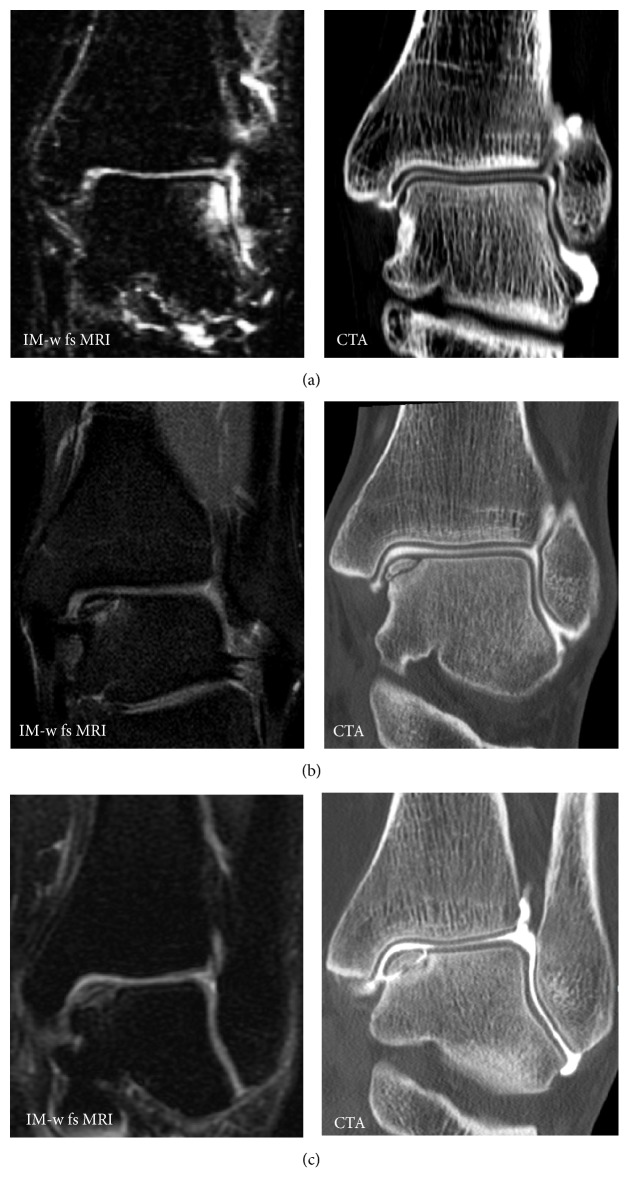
Different presentations of osteochondral defects. (a) Patient with suspicion of an osteochondral defect on MRI but no defect on CTA. (b) Patient with an osteochondral defect without loosening (no subsequent surgery). (c) Patient with similar findings on MRI as in (b); however on CTA contrast enhanced fluid surrounds the osteochondral fragment indicating instability and the patient had to undergo subsequent surgery.
